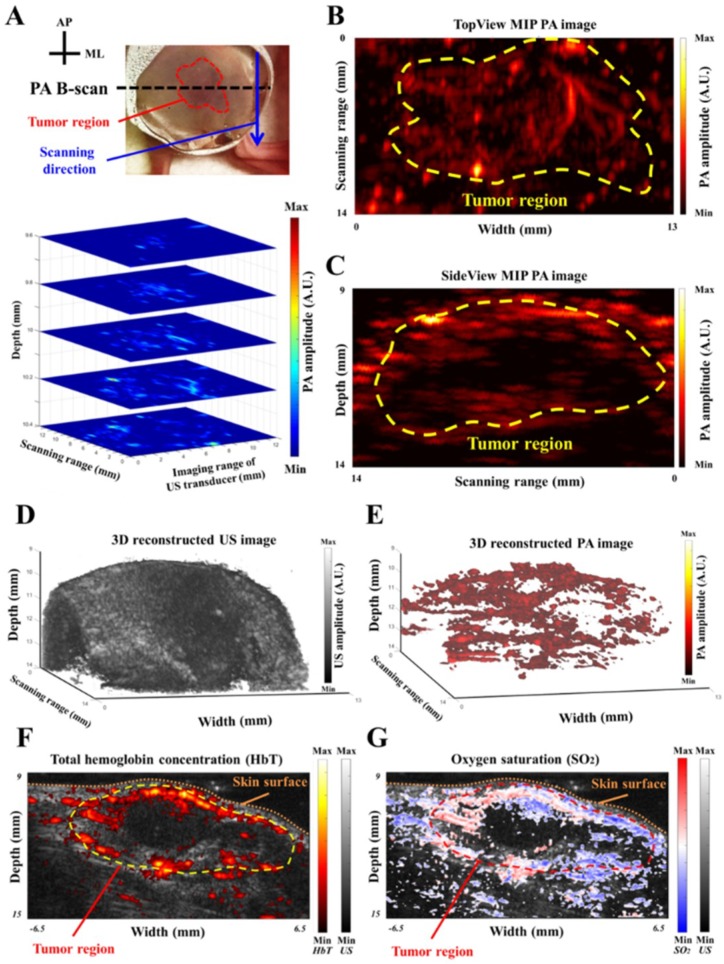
Subcutaneous tumor evaluation using the developed PA imaging system. (A) The tumor in the right hind leg was imaged by our PA imaging system. The upper subfigure shows that the tumor region (indicated by red dashed line) was scanned in the scanning direction (blue arrow) to acquire multiple X-Z plane PA B-scan images. The lower subfigure demonstrates the reconstructed 5-slice CBV C-scan images in the X-Y plane at different depths. (B,C) The TopView (X-Y plane) and SideView (Y-Z plane) MIP images of the subcutaneous tumor, respectively. Tumor regions are indicated by yellow dashed lines. (D,E) 3D reconstructed US and PA images of the subcutaneous tumor, respectively. (F) A normalized total hemoglobin concentration (HbT)-ultrasound (US) co-registered X-Z plane B-scan image. The distribution of HbT is highly correlated with the subcutaneous tumor profile acquired by US imaging. (G) A normalized oxygen saturation (SO_2_) image of the subcutaneous tumor. Note that the yellow and red dashed lines indicate the tumor regions in (F) and (G), respectively.
